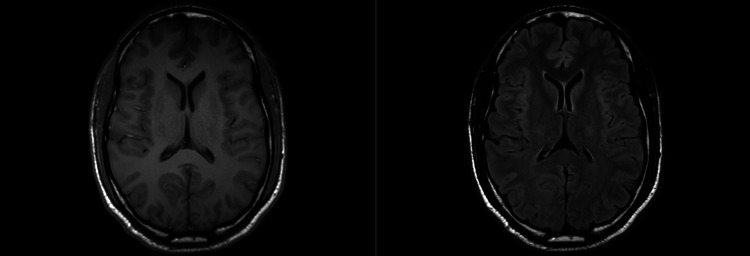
Unremarkable MRI of the brain
